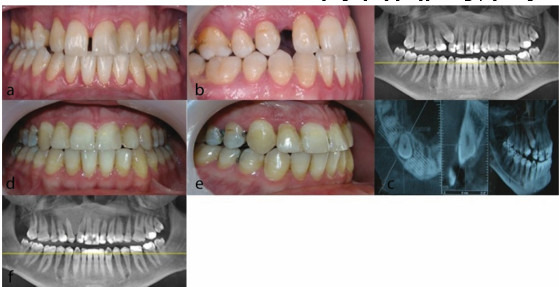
Patient 2. a: Frontal occlusion photograph before treatment, b: Lateral occlusion photograph before treatment, c: Panoramic and cone beam computed tomography (CBCT) views, d: Frontal occlusion photograph after treatment, e: Lateral occlusion photograph after treatment, f: Post treatment panoramic (total treatment time: 18 months)
